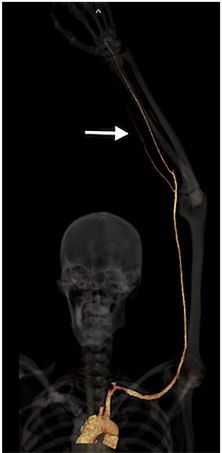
The image depicts the computed tomography angiography reconstruction with bone shadow. The white arrow demonstrates the vascular cutoff of the radial artery in the area of the soft-tissue laceration.
